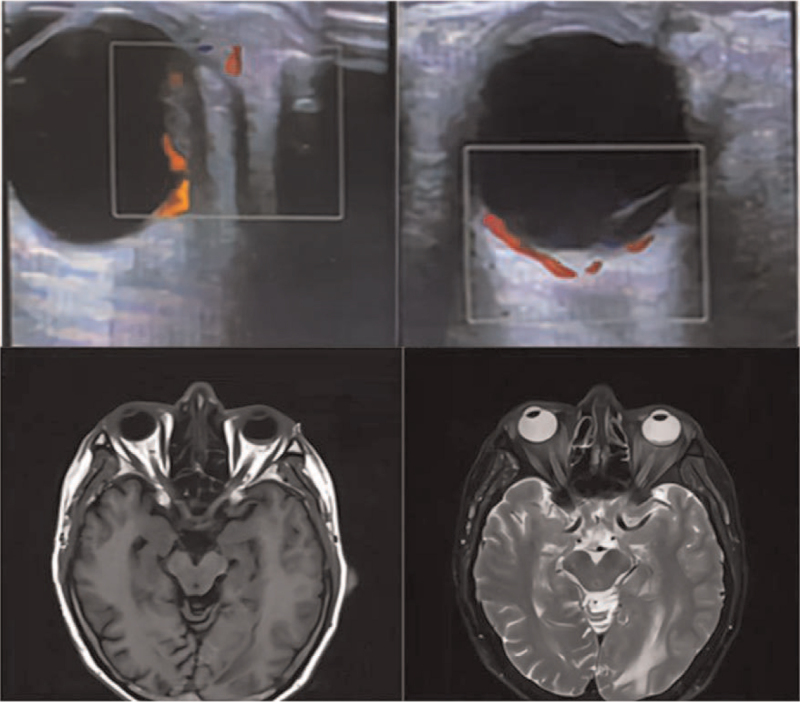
A strong echo of 10 × 4 mm was seen on the temporal side of the vitreous of the left eye and blood flow signals were seen inside. Vitreous membranous echo, no obvious signal inside. MRI: Irregular T1-weighted and T1-weighted low signal shadows were found in the posterior and lateral walls of the left eyeball, protruding into the eyeball.
